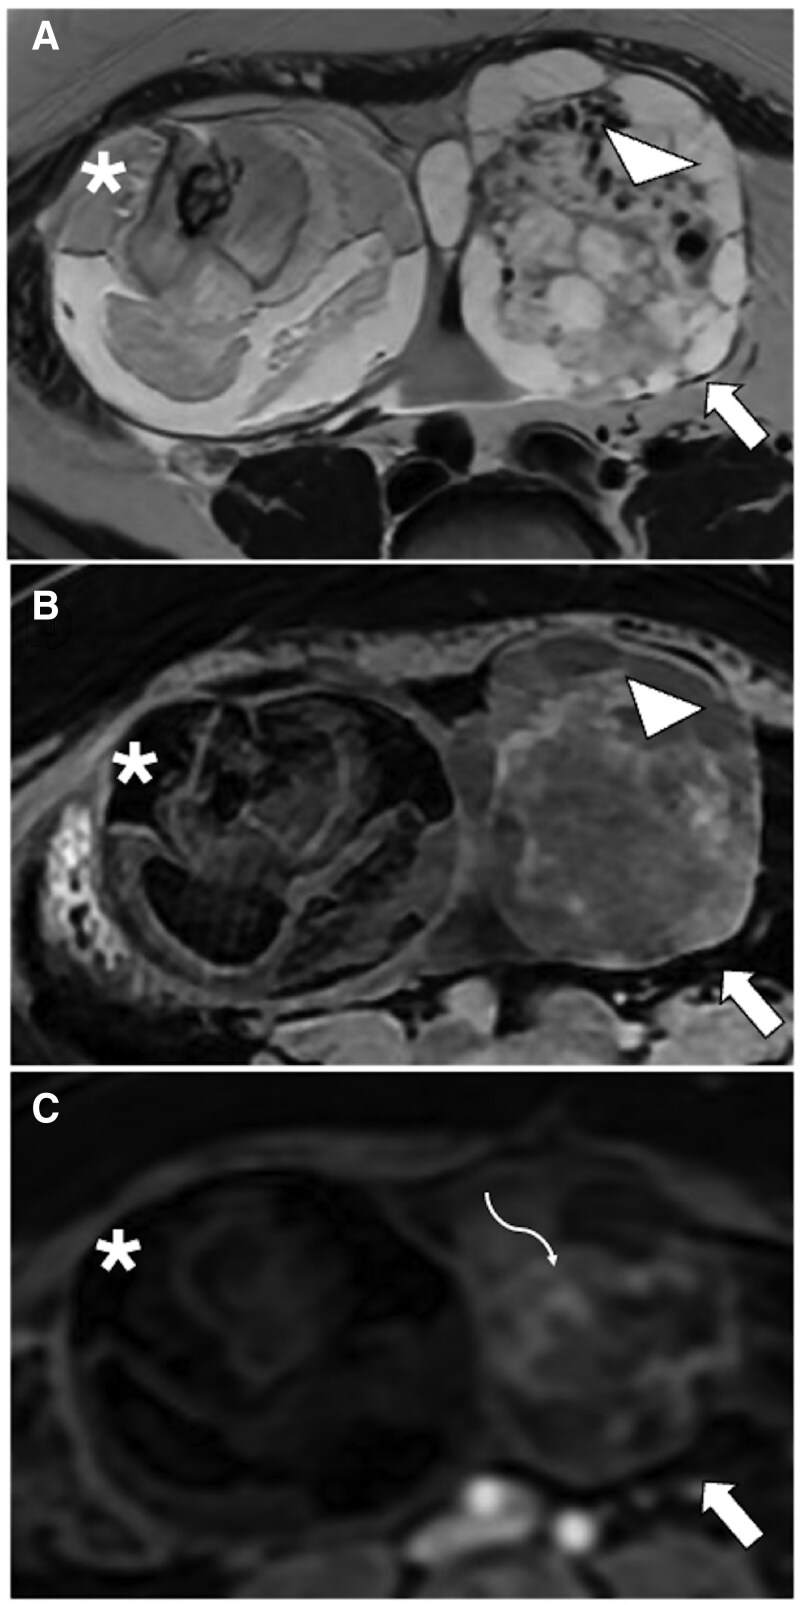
Struma ovarii. Struma ovarii typically presents as large multilocular lesion with loculi of variable SI on MRI. Some of the loculi (arrowheads in A and B) show low SI on T2-WI (A) and high SI on T1-WI (B) due to the colloid component. Vivid enhancement of the solid component is also visible (curved arrow) in the postcontrast T1-WI (C). A mature cystic teratoma can be seen on the right side of the struma ovarii (asterisk). Abbreviations: SI = signal intensity; WI = weighted imaging.
